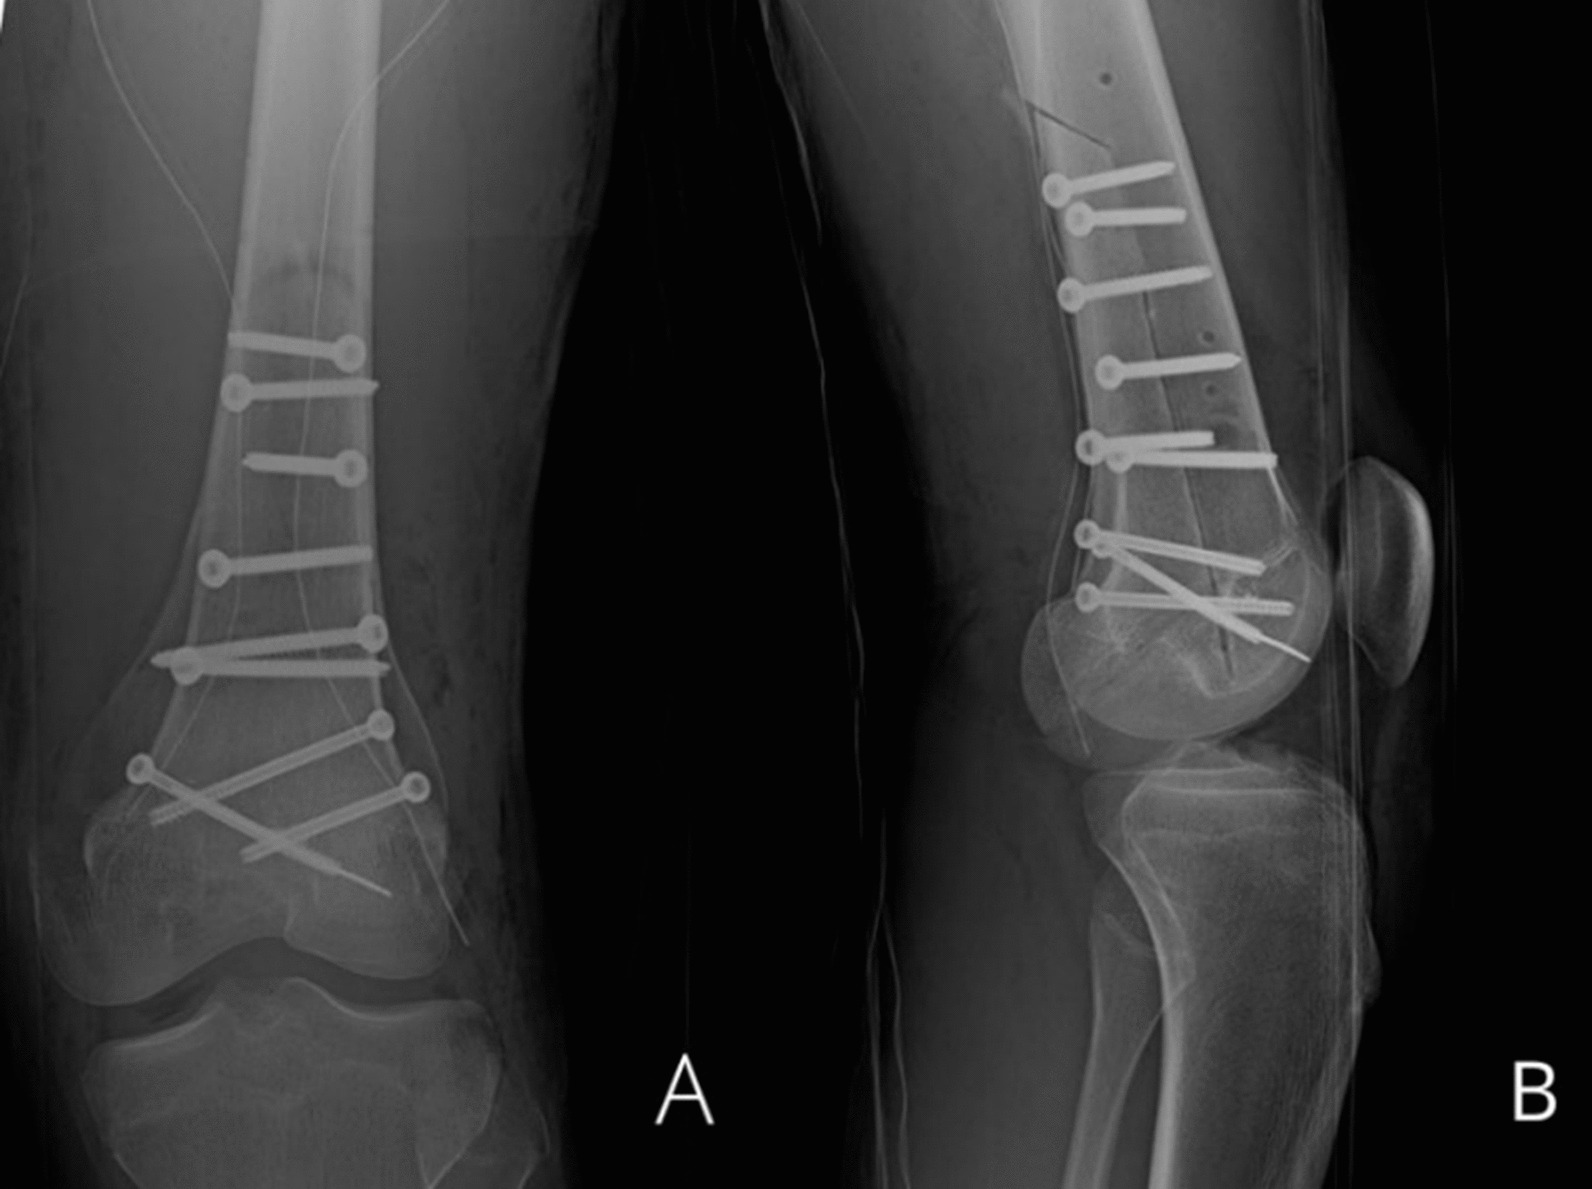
Antero-Posterior and Lateral A, B postoperative radiographs
